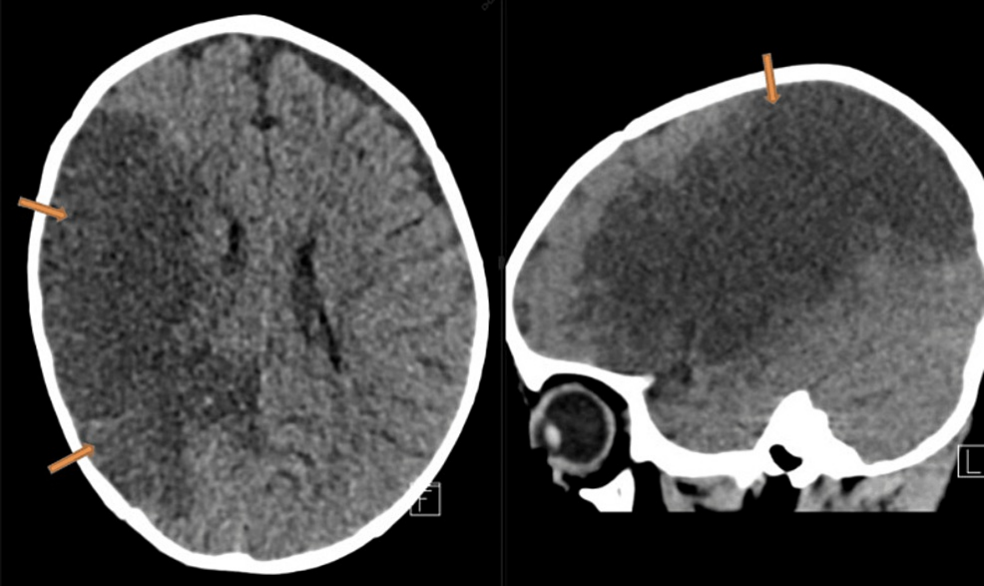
Selected axial image and sagittal multiplanar reconstructed image of a non-enhanced computed tomography of the brain shows evidence of a large area of hypoattenuation in the expected area of the right middle cerebral artery territory resulting in loss of gray-white matter differentiation.
